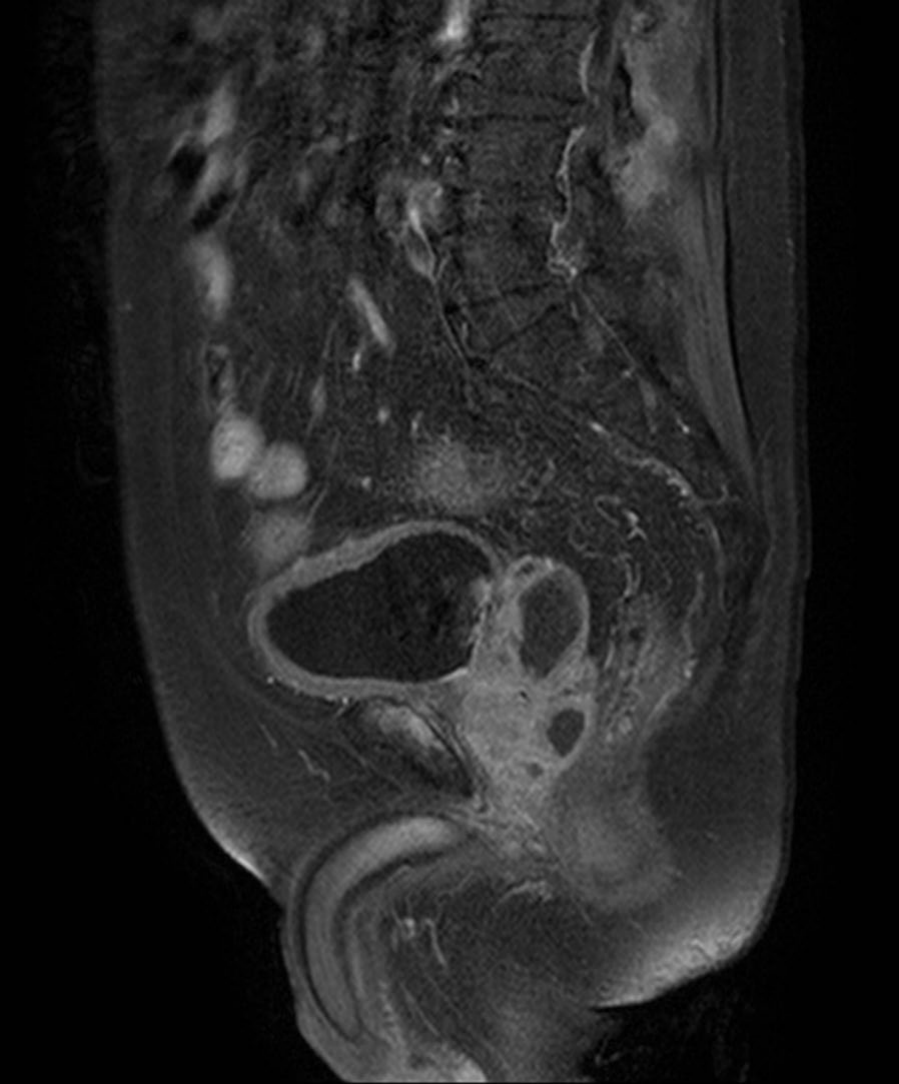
Fat suppressed MRI of pelvis denoted a hyperintensity lesion (*white arrow*) over the right pelvis below the bladder wall compatible with osteomyelietis
